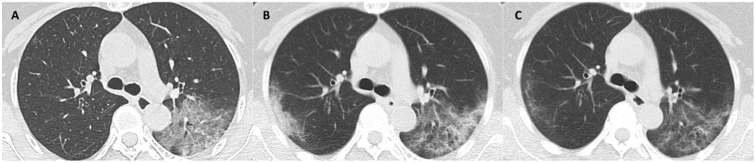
CT scans at 3 milestones in a 65-year-old woman with COVID-19 pneumonia. (A) Initial CT obtained on illness days 1 showed ground-glass opacity in left upper lobe. (B) Worst CT obtained on illness days 8 showed ground-glass opacity and consolidation with increased extent in bilateral upper lobes. (C) CT scan before discharge obtained on illness days 18 showed substantial residual ground-glass opacity. The patient was discharged on illness days 19.
